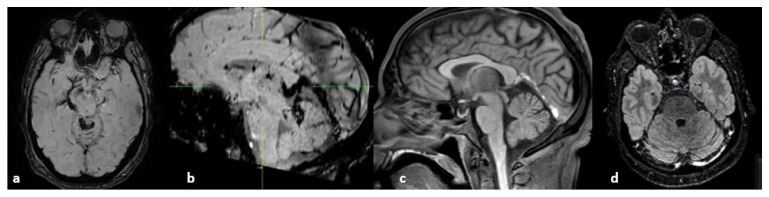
From left to right: (a,b) susceptibility-weighted imaging, axial (a) and sagittal (b) planes (thrombosis of the internal cerebral and basilar veins and straight sinus); (c) T1 sequence sagittal plane (spontaneous hypersignal of the thrombus in the straight sinus); (d) FLAIR image, axial plane (spontaneous hypersignal of the thrombus in the left lateral sinus).
